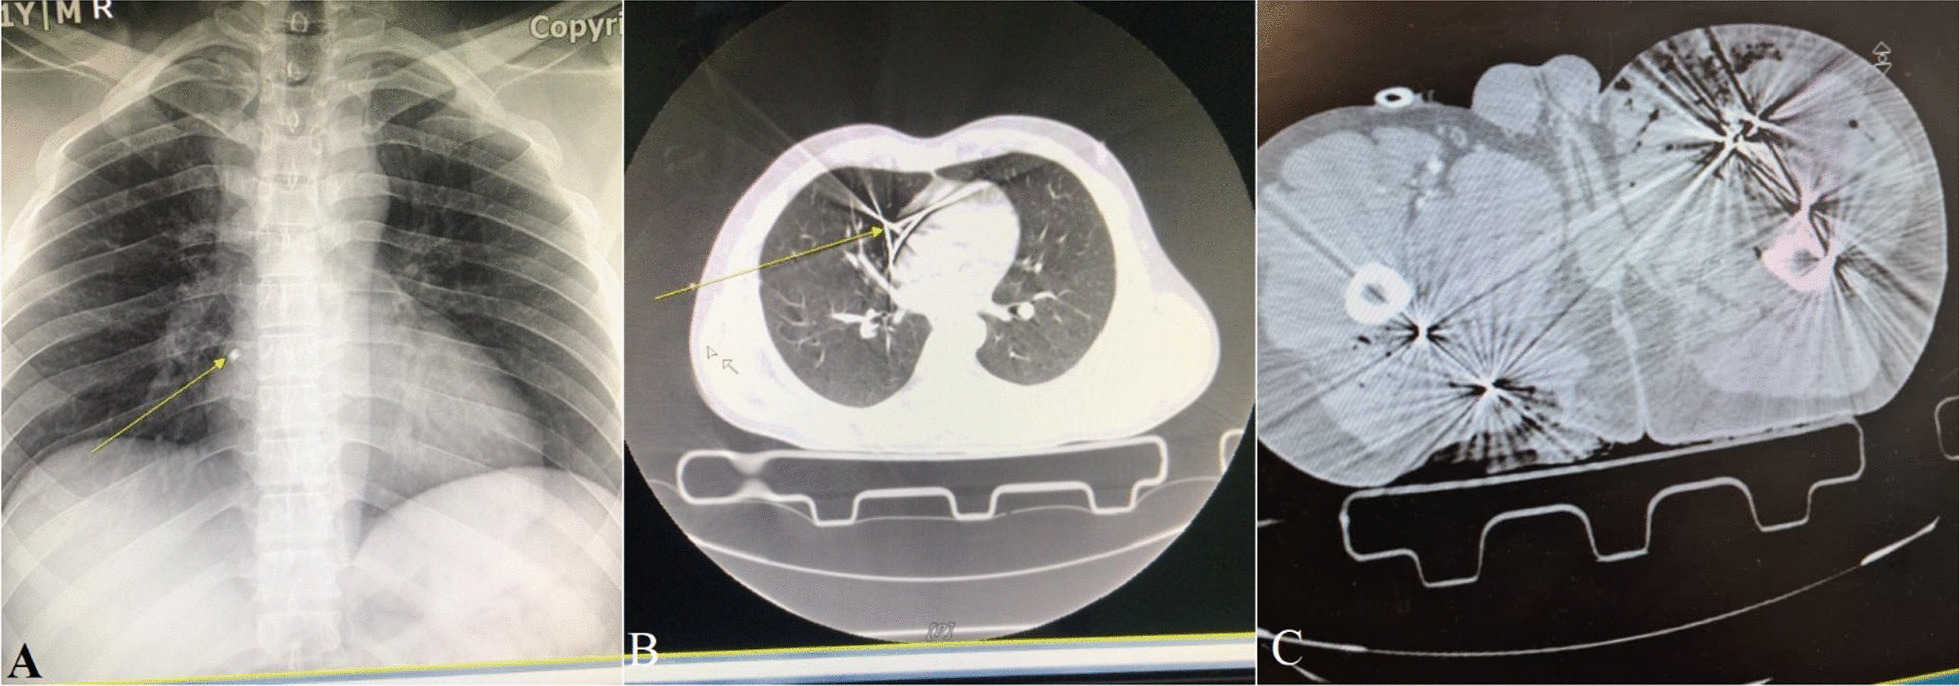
Radiological evaluation of the patient. (A) Chest X-ray at 1 day after surgery, demonstrating bullet pellet in the right atrium (yellow indicator). B Chest computed tomography scan demonstrating bullet in the branch of the right pulmonary artery (yellow indicator). C Lower-extremity computed tomography scan demonstrating bilateral shot gun injury to both gluteal and posterior thighs
